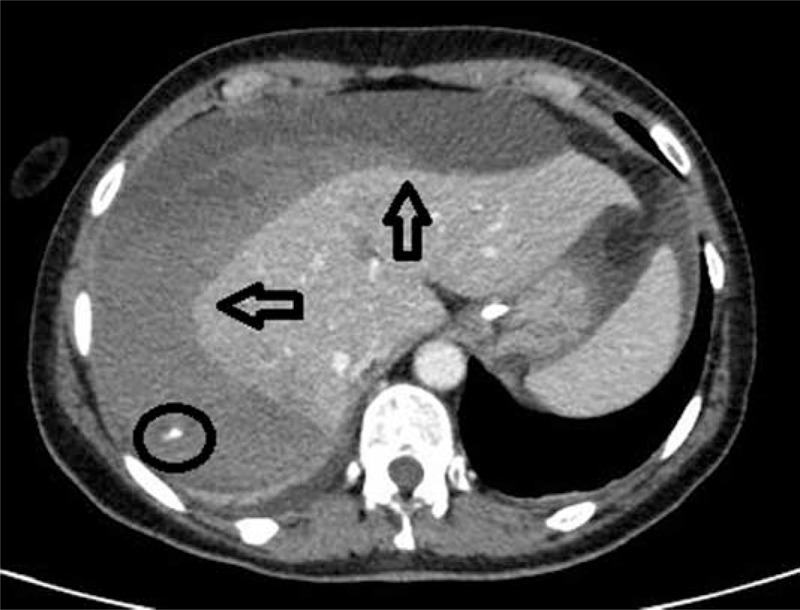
CT scan of upper abdomen showing a subcapsular hepatic hematoma (hypodense area indicated by the arrows) in which is present a source of active bleeding (circle). CT = computed tomography.
